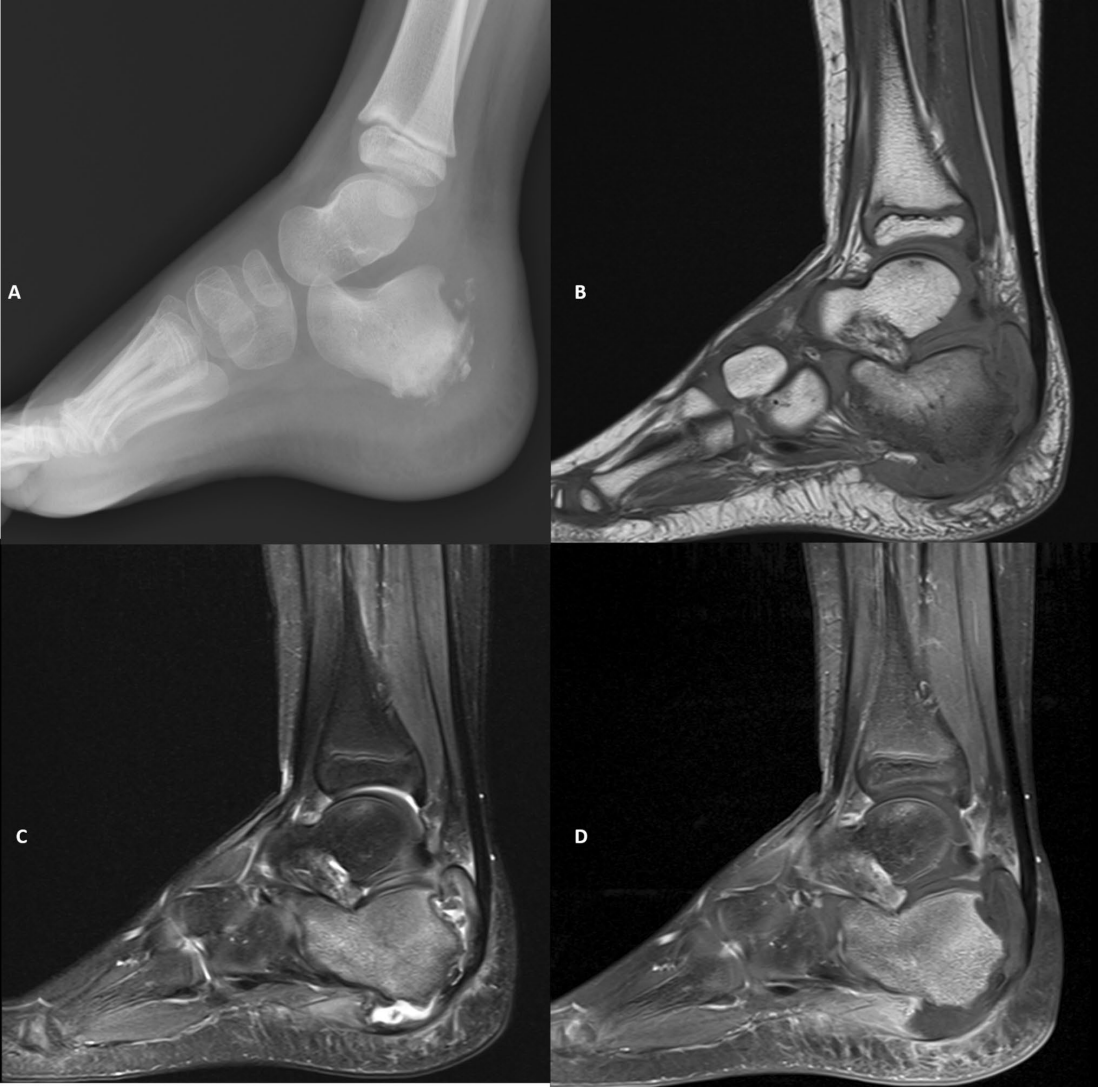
Lateral radiograph of the hindfoot at presentation (A) showing a sclerotic remodelling of the posterior calcaneal body with sclerosis and fragmentation of the apophysis, retrocalcaneal scalloping and moderate associated soft tissue swelling. Further evaluation with MRI with sagittal T1 and T2 weighted imaging reveals loss of normal T1 marrow signal (B) at the posterior calcaneus with diffuse marrow oedema (C) and associated contrast enhancement on post-gadolinium sequences (D). Soft tissue collection involving the fragmented calcaneal apophysis with a non-enhancing and low T1/T2 signal margin (B–D). Modest surrounding soft tissue oedema and enhancement at the hindfoot region
